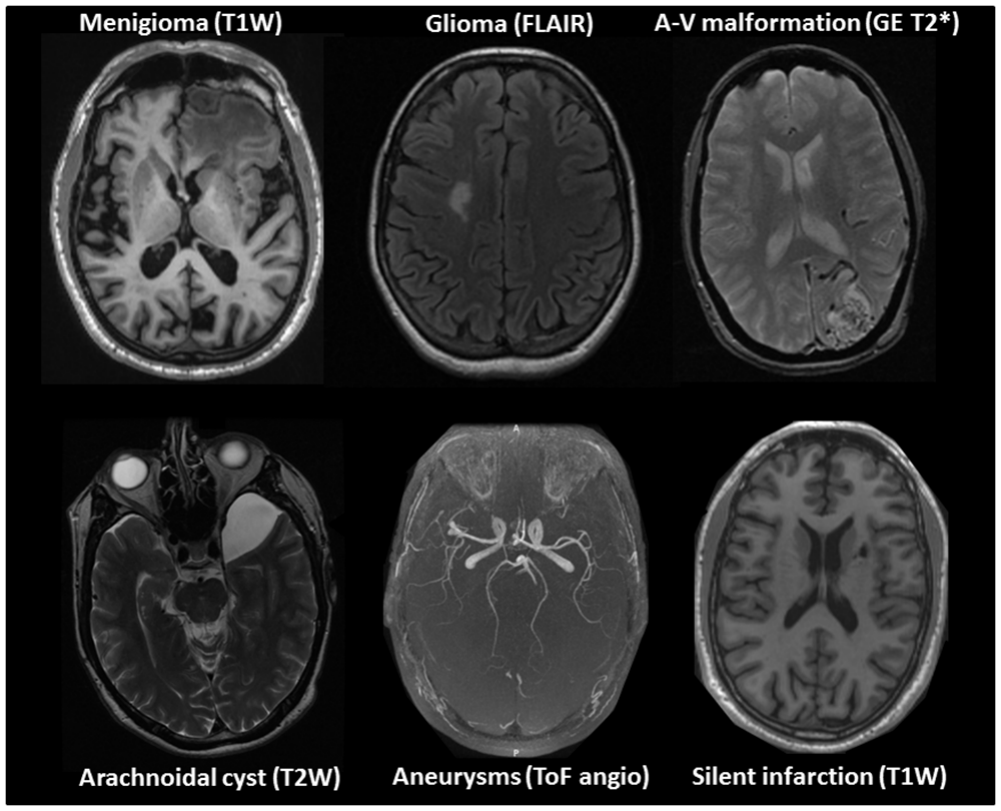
Examples of the different scan sequences and incidental intracranial findings in the HUNT MRI cohort, i.e. meningioma (T1W 3D), glioma (FLAIR), arteriovenous malformation (T2* weighted), arachnoidal cyst (T2W weighted), aneurysms (Time of Flight angio) and silent infarctions (T1W 3D scan).
